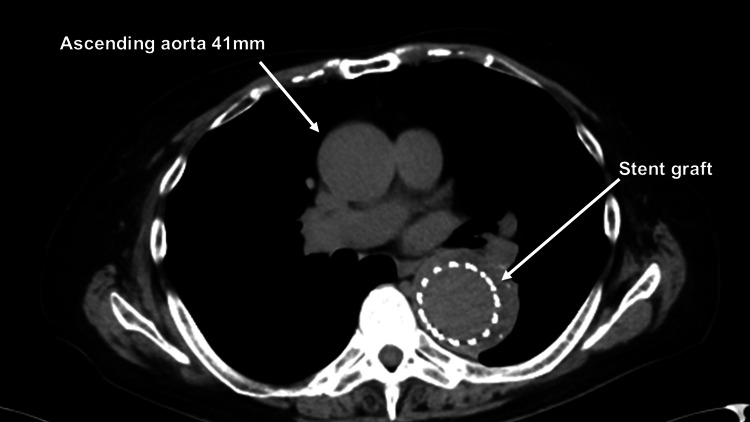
Computed tomography nine months earlier A computed tomography scan nine months earlier revealed that the diameter of the ascending aorta is 41 mm.
